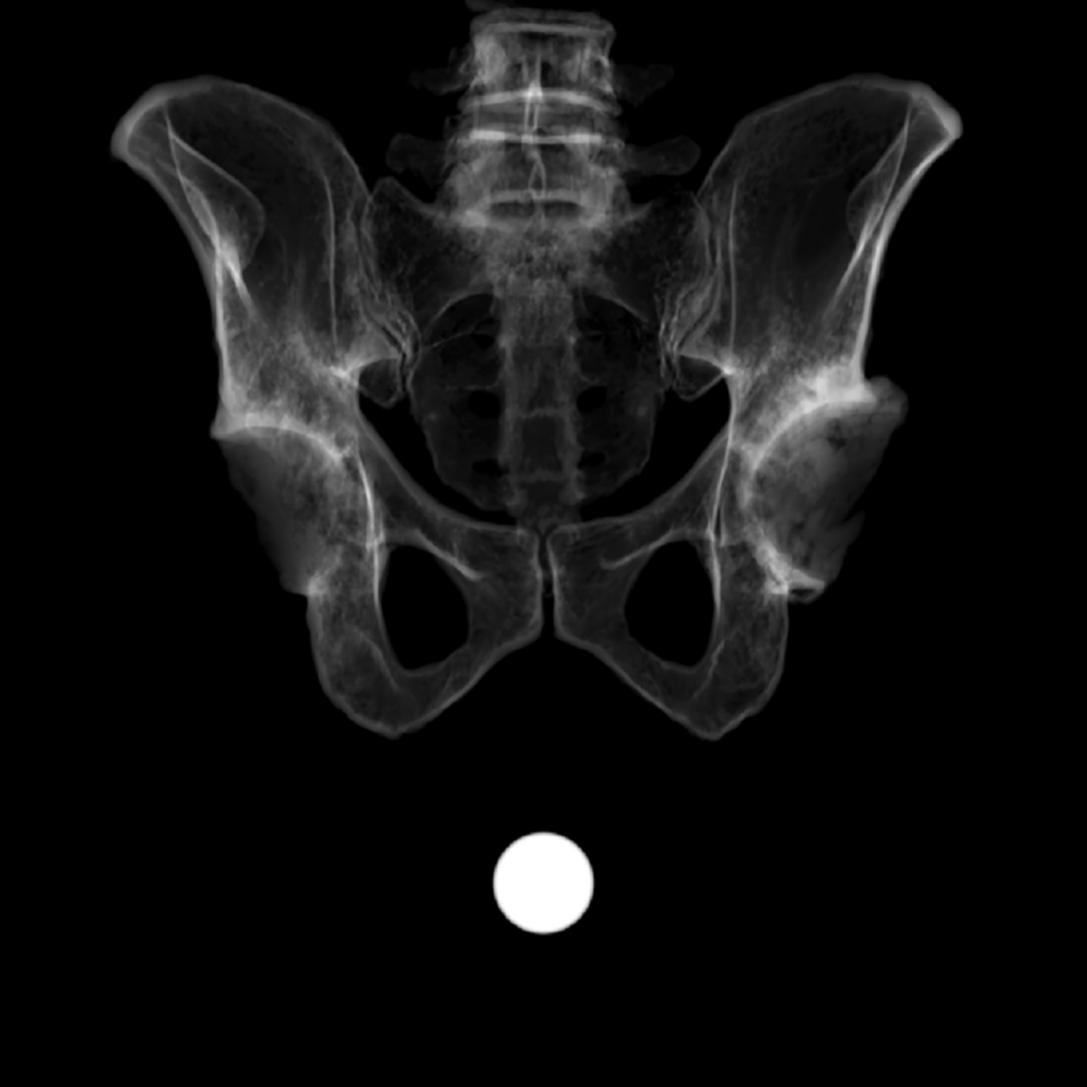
Synthetic radiograph created from 3D rendering; the 25.4 mm scaling ball is visible in the image
